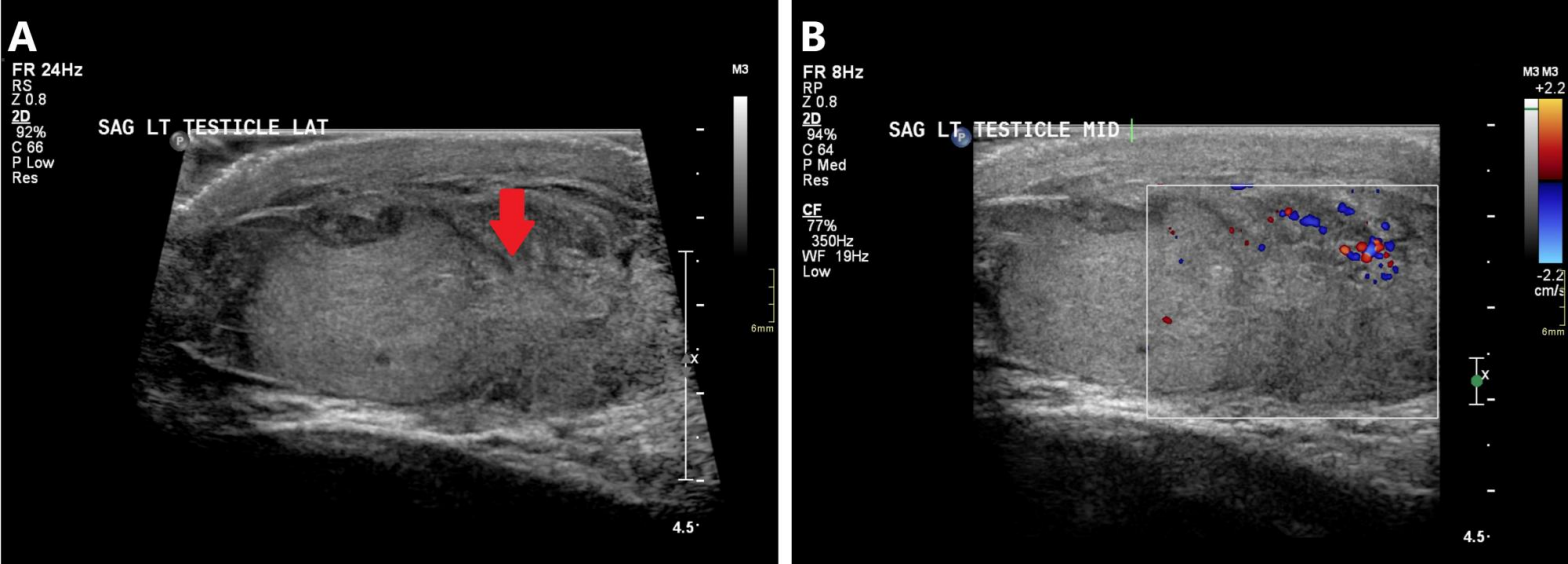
Left testicular rupture. (A) There is a disruption of the tunica albuginea (red arrow) with a loss of the normal ovoid shape of the left testis. (B) Doppler interrogation shows relative avascularity in the surrounding heterogeneous collection consistent with a hematocele.
